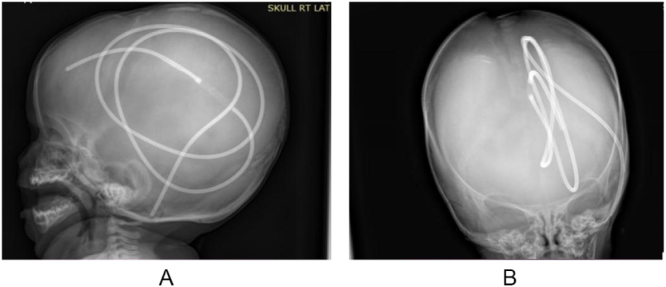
Lateral (A) and anteroposterior (B) skull X-ray images reveal migration of both proximal and distal segments of the ventriculoperitoneal shunt into the intracranial cavity with no disconnection between the two segments.
